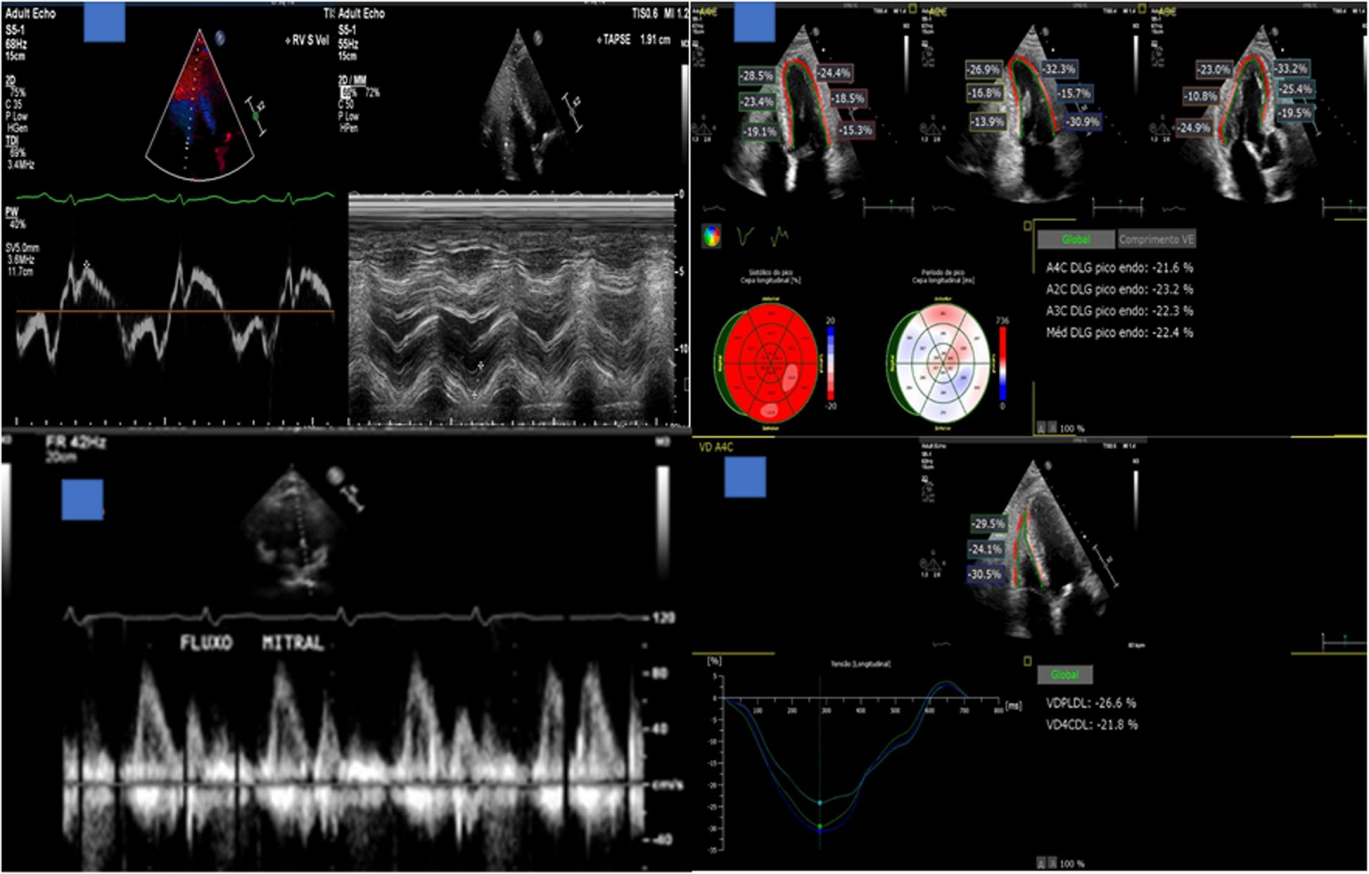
Images of the complete echocardiographic protocol of the investigation
